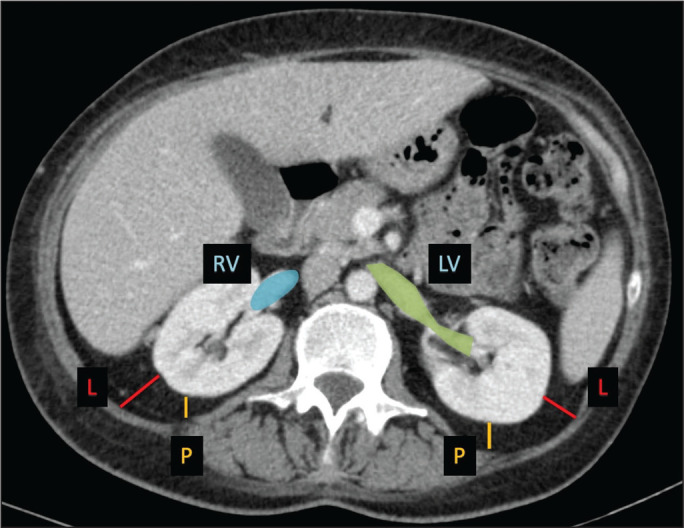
Axial CT scan demonstrating the method of measuring perinephric fat at the level of the renal vein to determine the MAPS. RV, right (renal) vein; LV, left (renal) vein; L, lateral; P, posterior.
